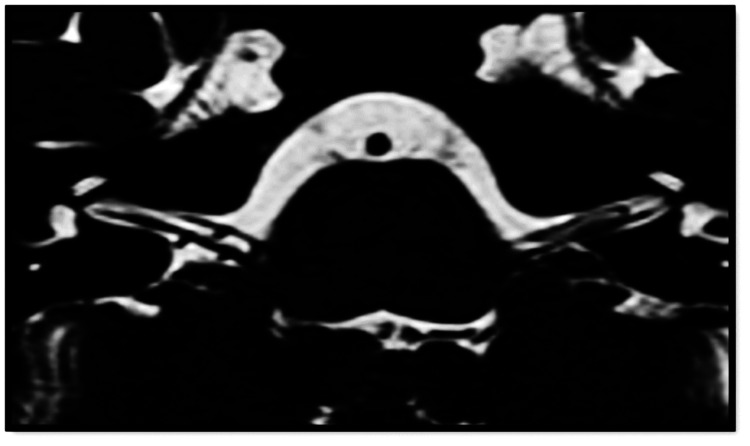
Chavda type III vascular loop. Axial high-resolution T2-weighted 3D-CISS sequence demonstrates the left AICA loop extending into the IAC (>50%). CISS = constructive interference in steady state; AICA = anterior inferior cerebellar artery; IAC = internal auditory canal
